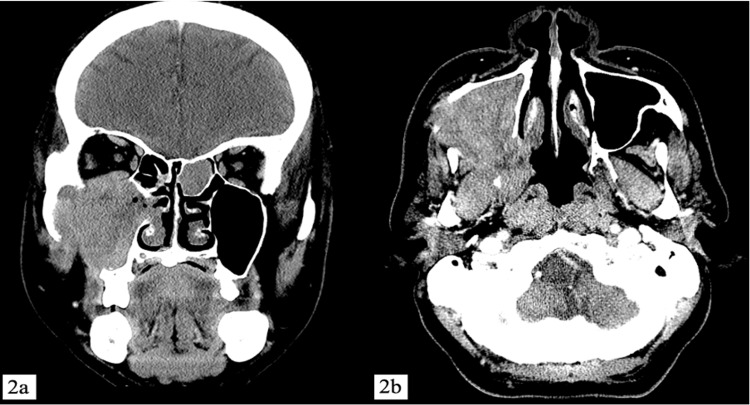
Contrast-enhanced computed tomography (CECT) of paranasal sinuses coronal (a) and axial (b) views CECT shows extensive hypodense mass occupying the whole of the right maxillary sinus with bony destruction and erosion of the right orbital floor, lateral wall of maxilla with extension to cheek.
